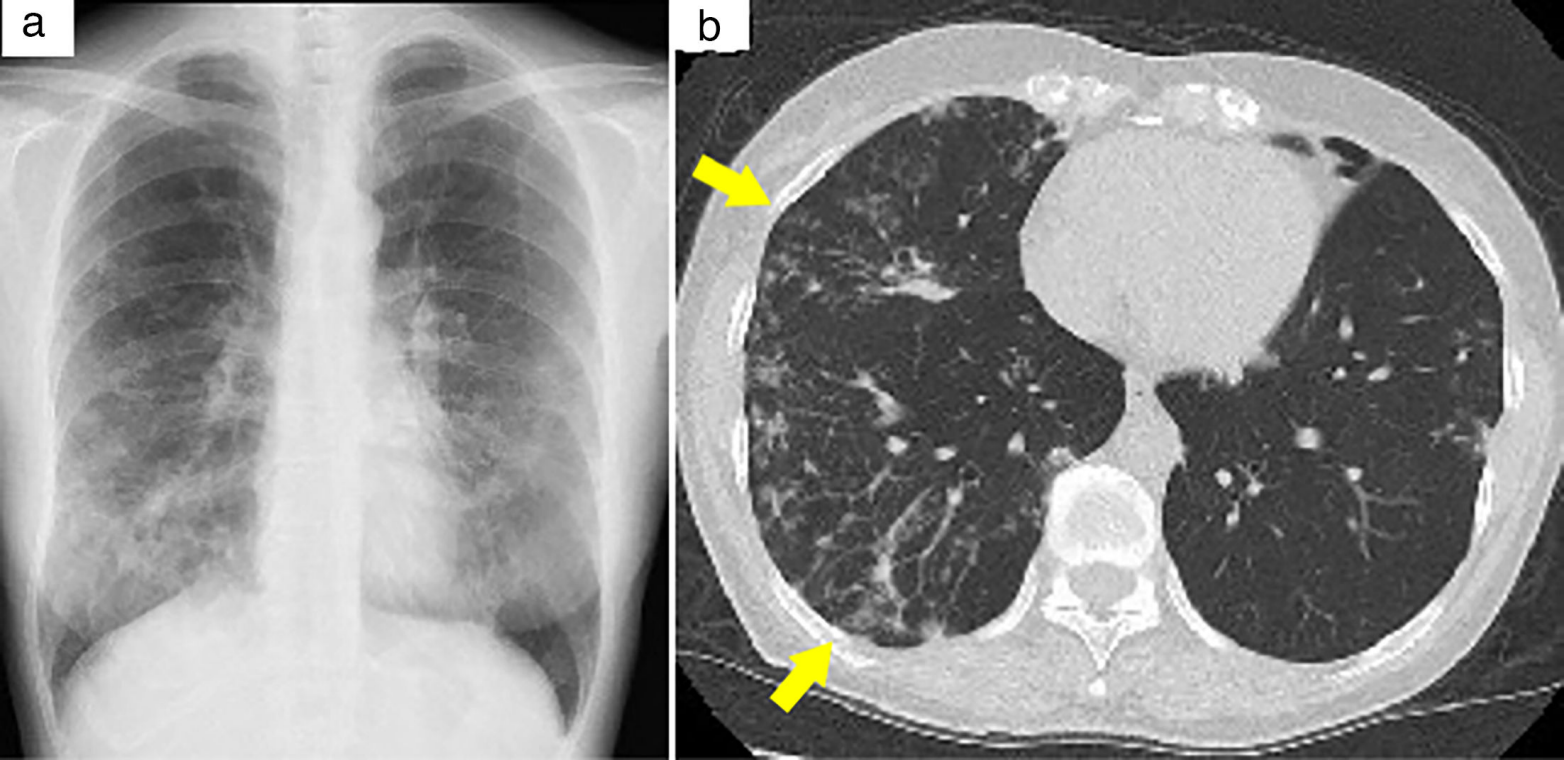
Chest radiography and high-resolution computed tomography (HRCT) at initial visit. (a) Chest radiograph and (b) HRCT image showing small centrilobular nodules, patchy consolidation, and bronchiectasis, suggesting a nodular bronchiectatic type pulmonary nontuberculous mycobacterial disease (arrows).
